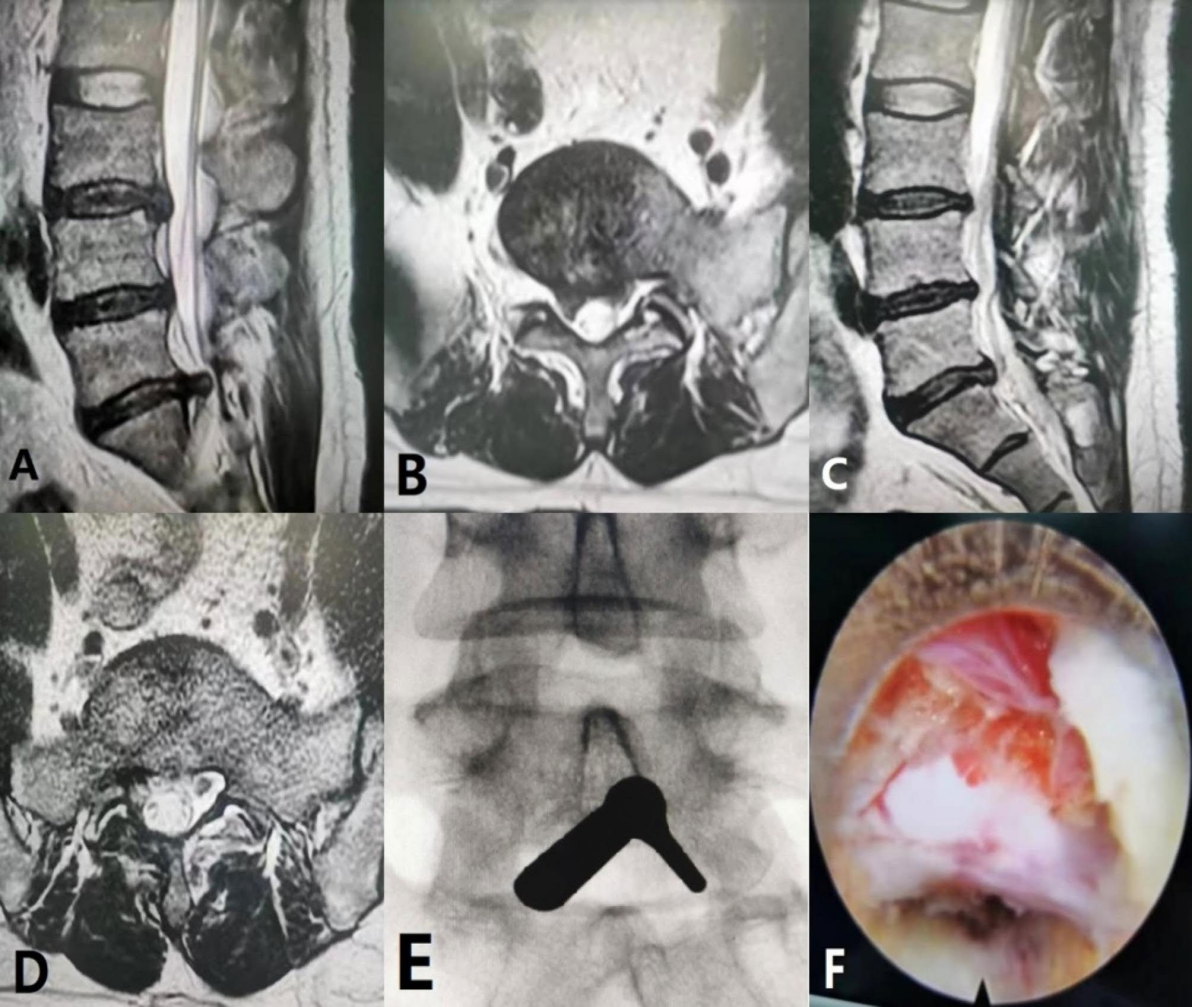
Images of a 40-year-old man who underwent intralaminar endoscopic lumbar discectomy. Preoperative T2-weighted sagittal (A) and axial (B) magnetic resonance images show an L5-S1 disc herniation. Sagittal (C) and axial (D) images 2 years after surgery show complete removal of the herniated portion of the disc. Intraoperative images show (E) the beveled working sheath at the interlaminar space and (F) the S1 nerve root after decompression
